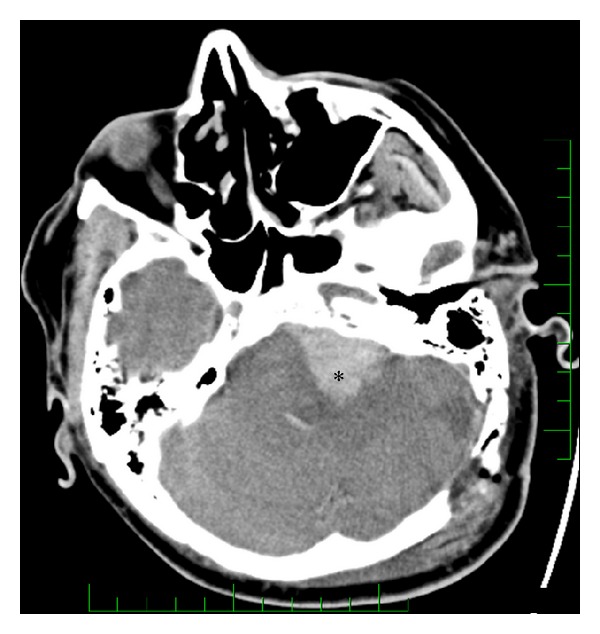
CT scan of patient with haematoma of the cerebellopontine angle.
